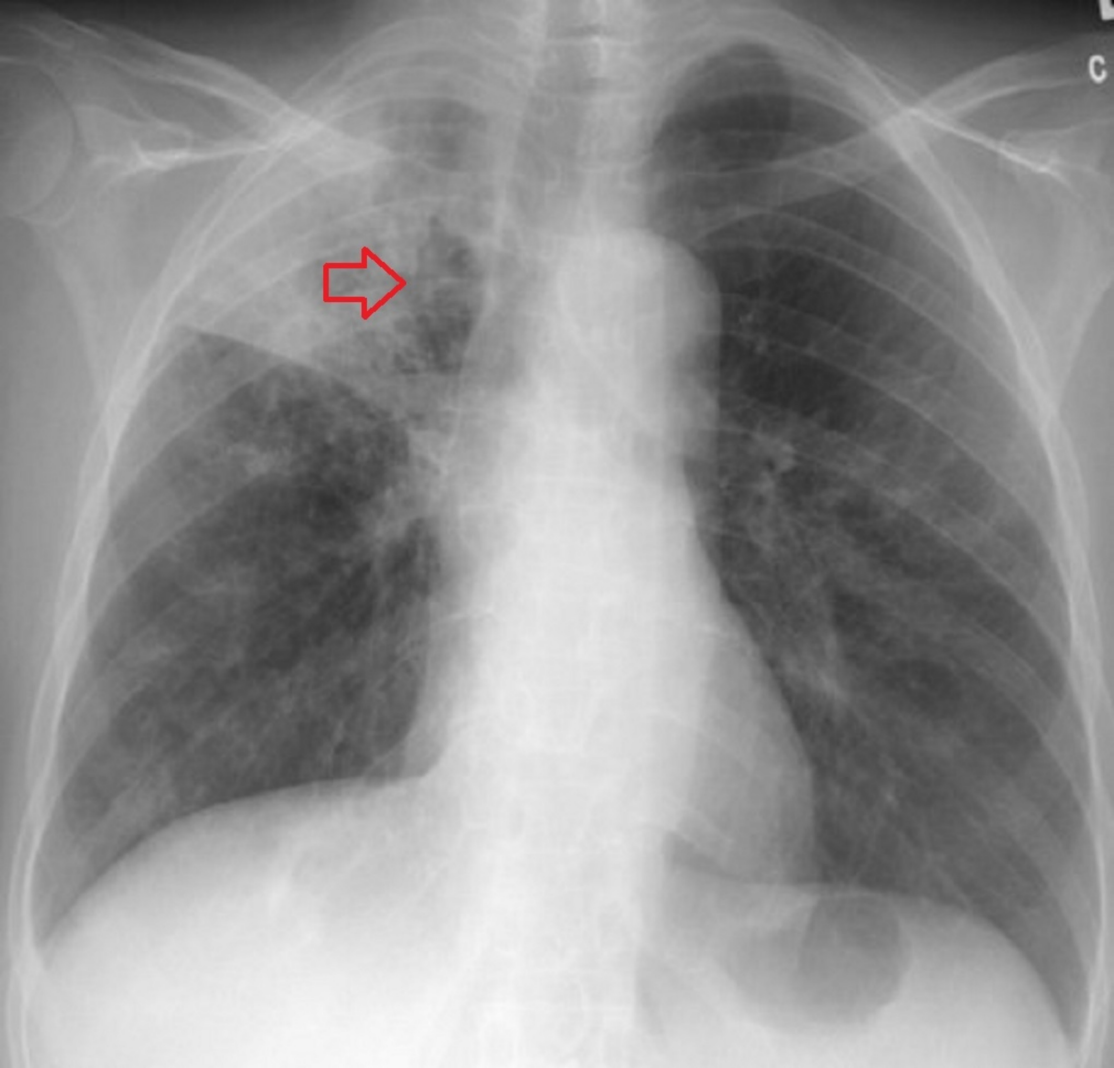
X-ray tuberculosis. Probable cavity in the right lung apex with evidence of consolidation.
